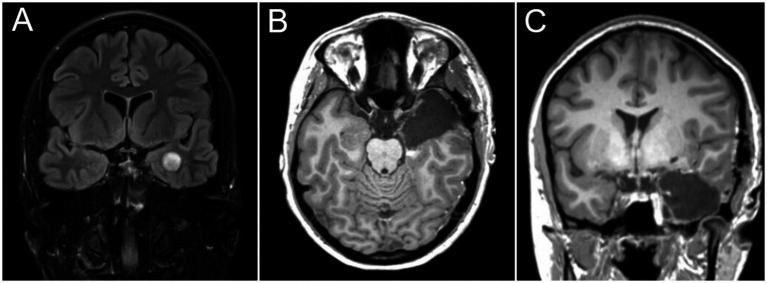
Surgical treatment (standard dominant-side anterior temporal lobectomy) for a ganglioglioma CNS WHO° I in a 22-year-old patient presenting with drug-resistant epilepsy. (A) Preoperative; (B,C) postoperative MRI scans.
